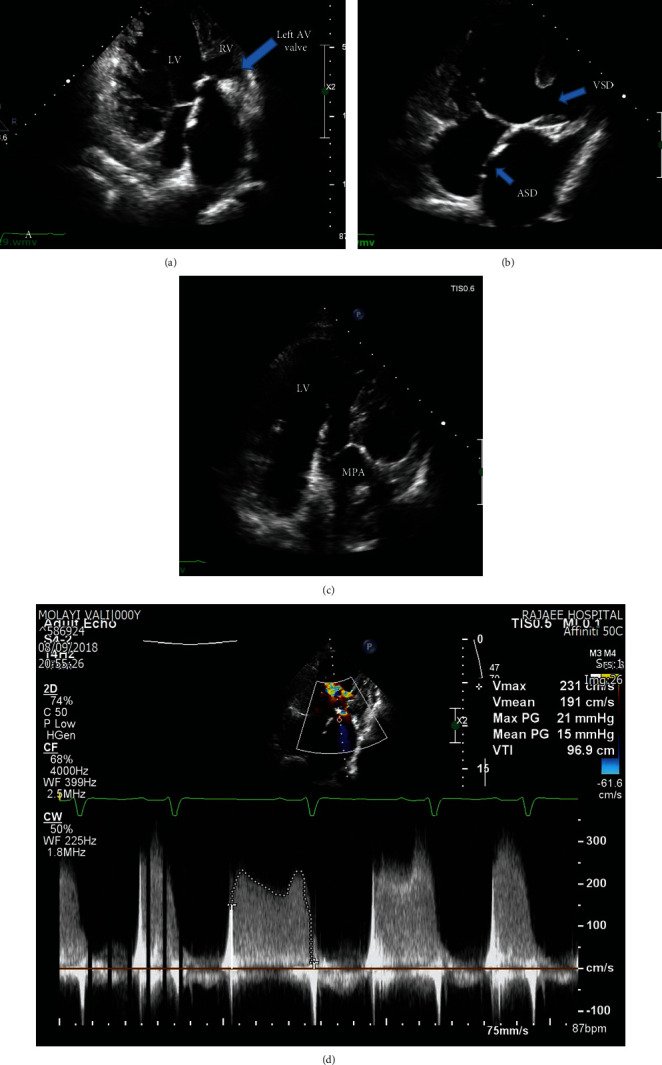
Transthoracic echocardiography in 4-chamber view shows (a) morphologic LV which is the right side of rudimentary RV, (b) large inlet type VSD, very small ASD, (C) PA originate from the main ventricle with confluent PABs, (d) Thick and hypo plastic left AV valve severe stenosis (MG:15, PG:21).
